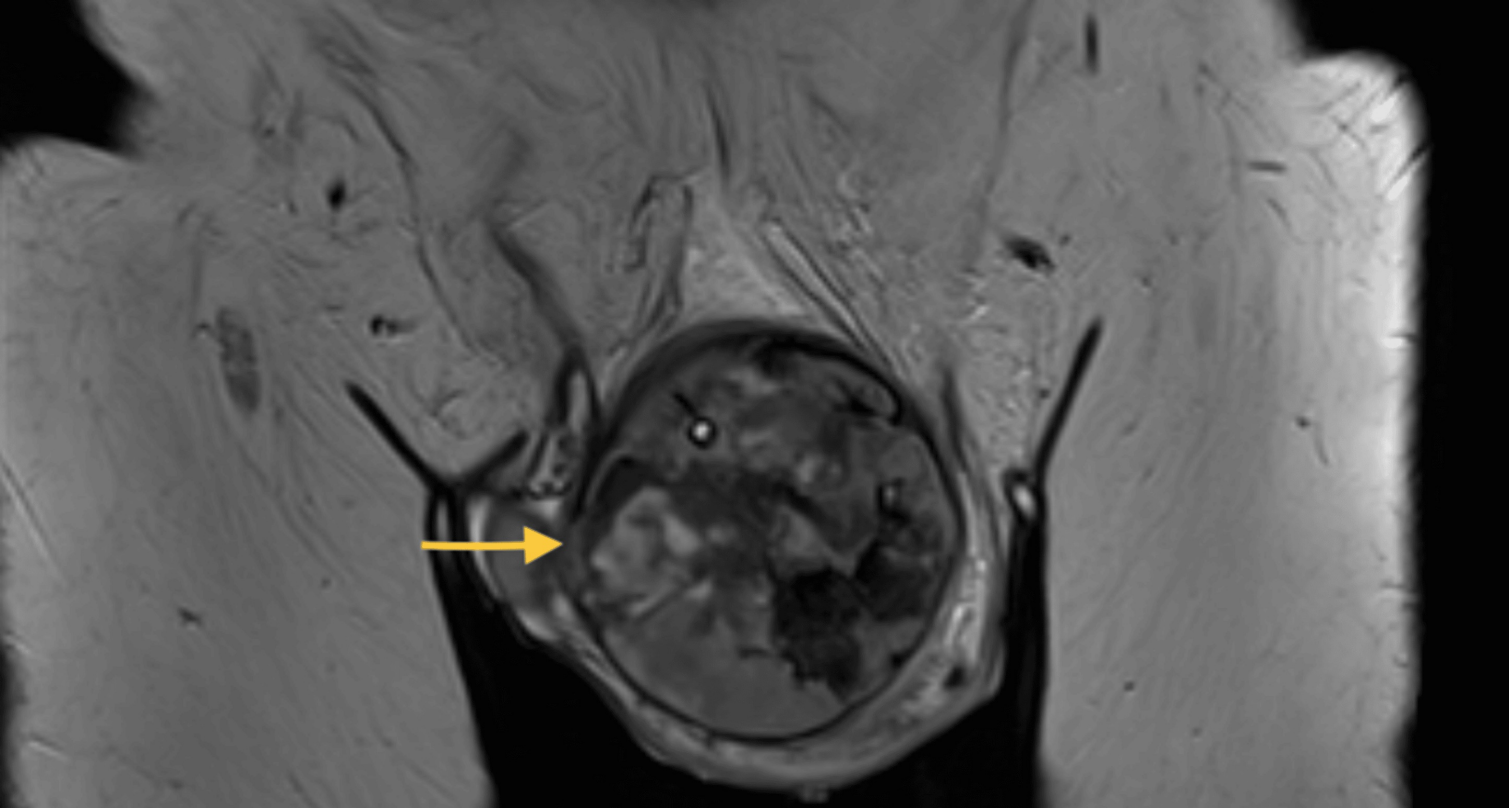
Coronal slices of MRI pelvis demonstrating malignant mass likely from penile cancer
